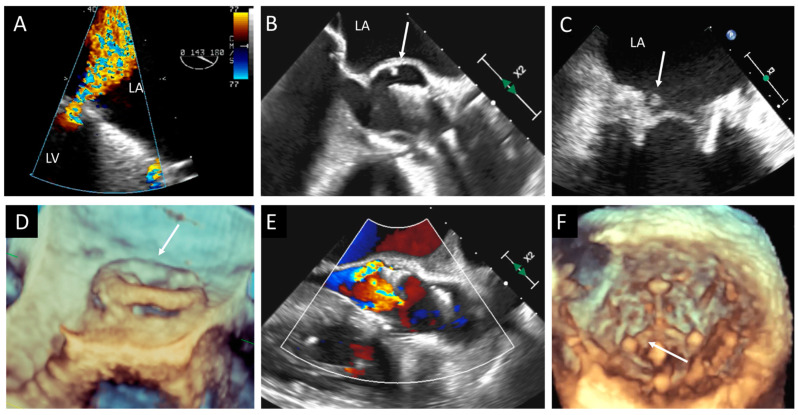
Three cases of prosthetic valve endocarditis: (A,D) dehiscence and para-prosthetic leak of a mitral valve prosthesis (arrow), (B,E) pseudoaneurysm (arrow) of an aortic prosthesis, and (C,F) small vegetation (arrow) in a prosthetic MV visualized in 2D TEE and confirmed in 3D TEE from LV view. LA, left atrium; LV, left ventricle; MV, mitral valve; TEE, transesophageal echocardiography.
